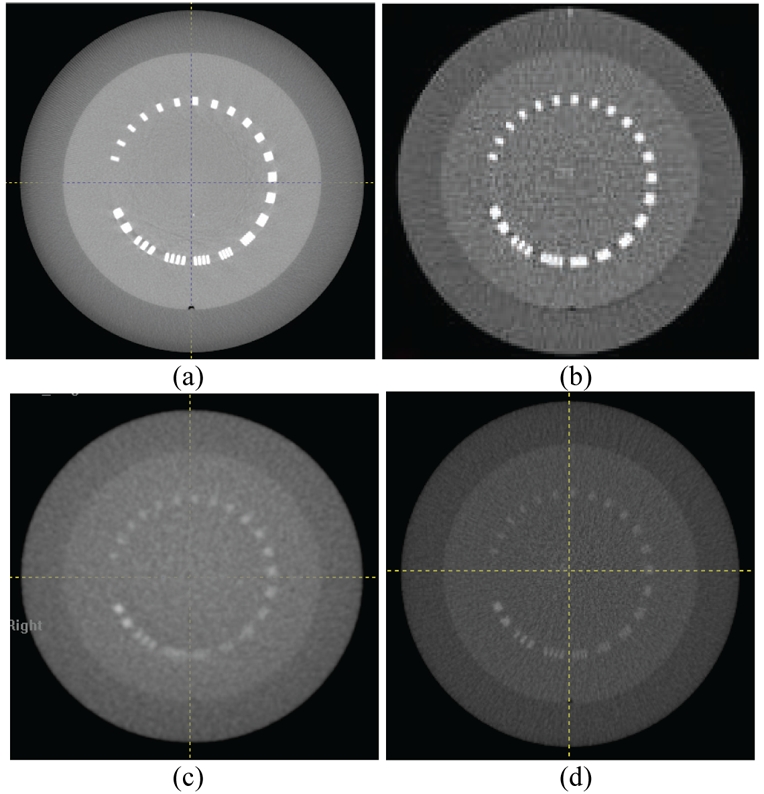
Images of CTP528 module of the Catphan acquired to determine spatial resolution with the four CBCT/FBCT systems; a) Varian kV CBCT; b) Elekta kV CBCT; c) Siemens MV CBCT; d) Tomo MV FBCT.
